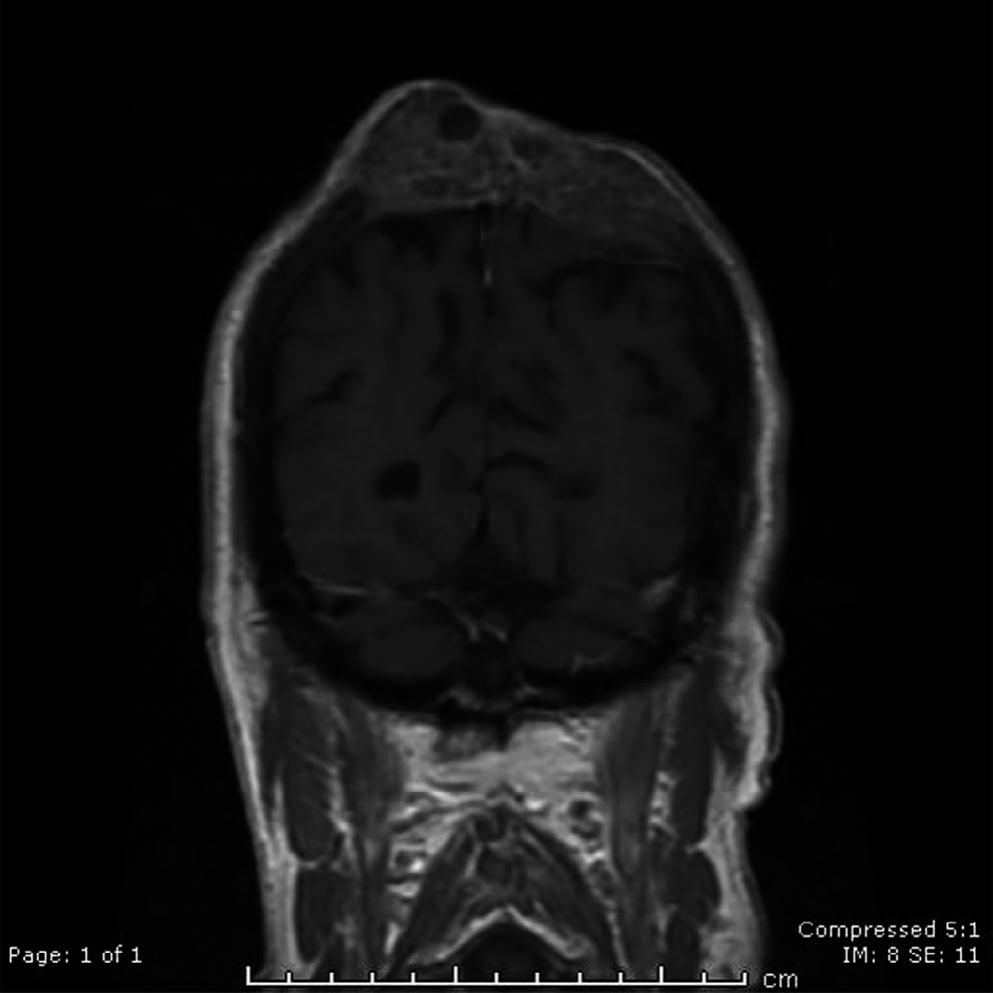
Coronal T1 post‐contrast MRI showing a large destructive, partly cystic mass lesion Involving the parietal bone with non‐homogenous contrast enhancement. *Refers to as an invasive disease, whereby cancer cells have grown beyond the epidermis.  Programmed cell death protein 1 on the surface of T and B cells in regulation of the immune system's response to cells of the human body
